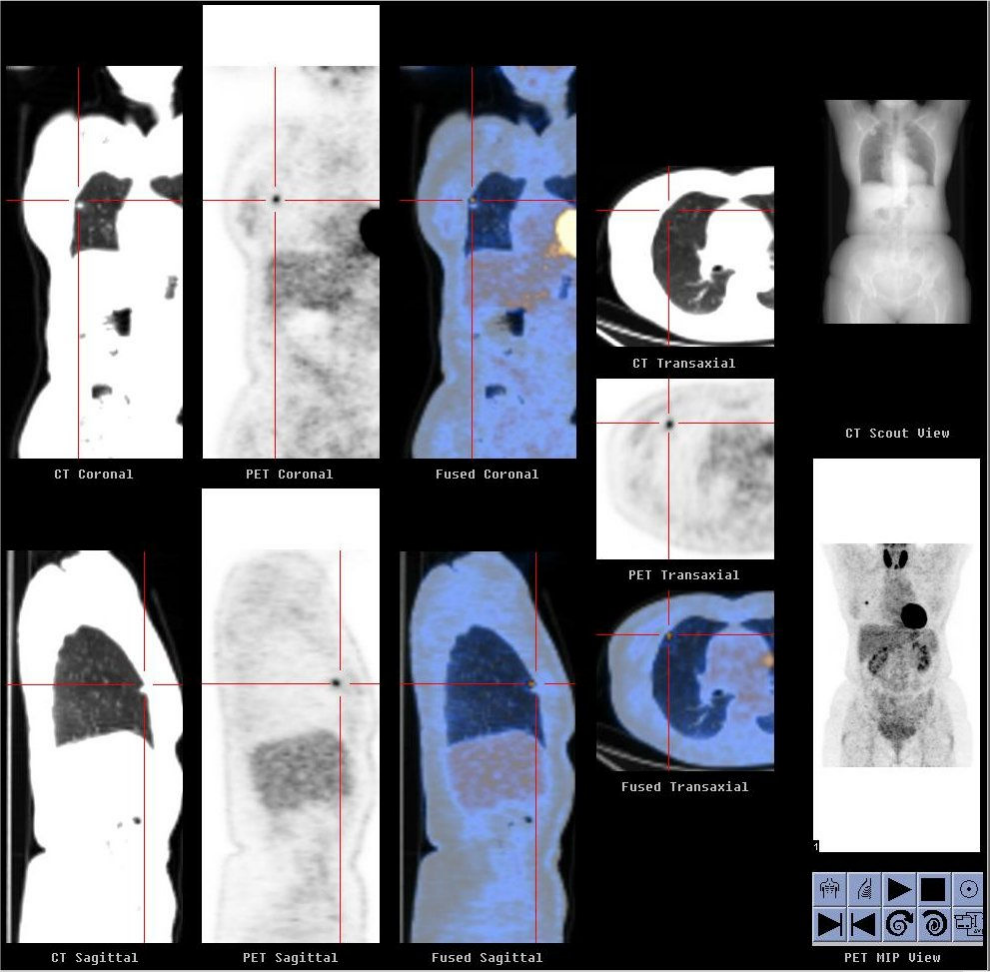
Misregistration of CT and PET malignant nodule of the right lung due to respiratory movement.
